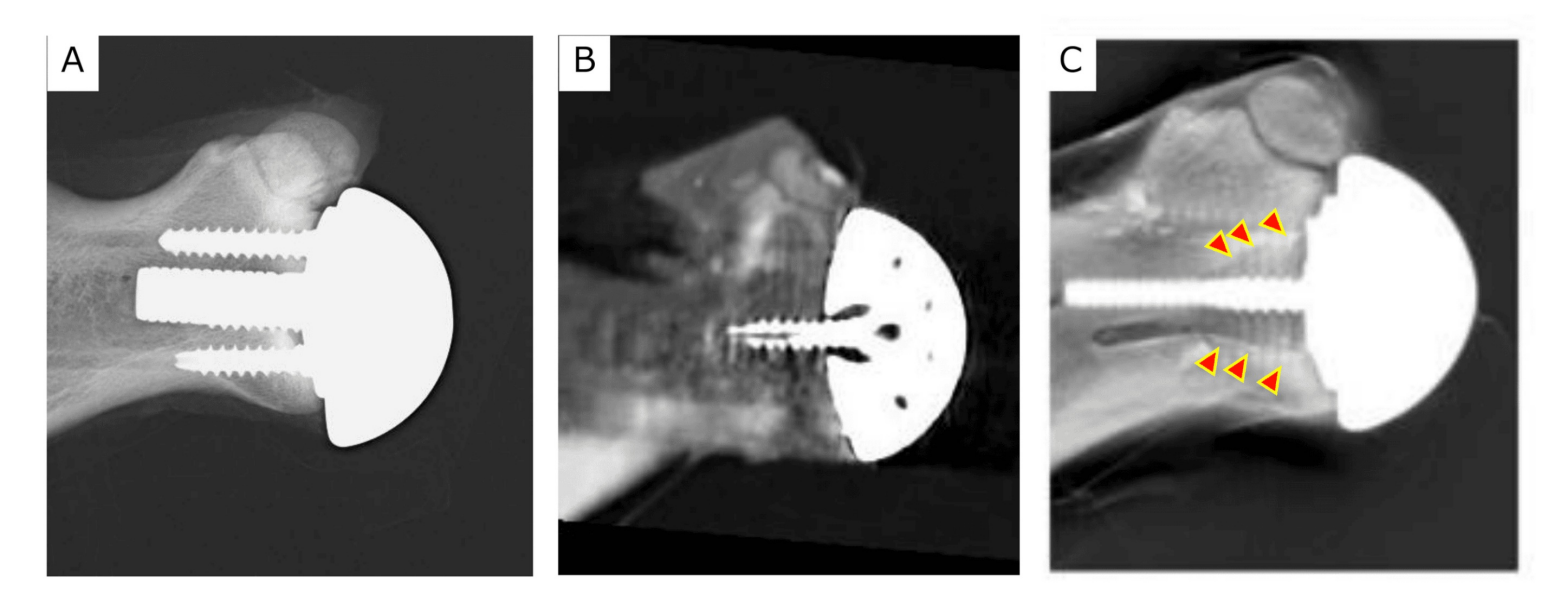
Posterior glenoid bone defect model a. radiographic evaluation, b. coronal CT image, and c. tomosynthesis. Arrowheads show areas of the posterior glenoid bone defect.
